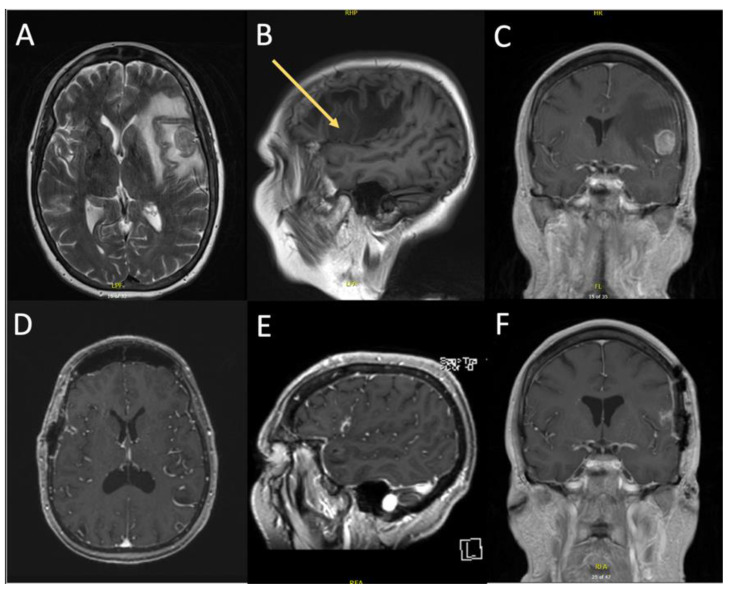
Preoperative MRI (top images) compared with 15-day postoperative MRI (bottom images). Image (A): Axial weighted T2 demonstrating extensive edema associated with lesion seen as a hyperintense signal with midline shift. Image (B): T1 MRI pre-Gd showing a hypointense, rounded, well-defined lesion within pars triangularis of the left inferior frontal gyrus (IFG). Vasogenic edema is visible as a hypointense signal. Image (C): T1-Weighted coronal view with contrast demostrating homogenous enhancement of the lesion and clear visualization of its margins. Ipsilateral collapsed lateral ventricle and midline shift secondary to surrounding hypointense edema is present. Image (D): Weighted T1 post-Gd with complete resection of the lesion. There is a small pneumocranium and a hyperintense signal beneath the craniotomy representing a small subarachnoid hemorrhage. Image (E): T1 weighted MRI showing a small hyperintense signal in the left IFG where the metastasis was previously located. Peritumoral edema is not present compared to before surgery. Image (F): Coronal weighted T1 post-Gd giving another view of the complete resection of the lesion with craniotomy, small hyperintense subarachnoid hemorrhage, and small pneumocranium. The previously collapsed left lateral ventricle is nearly symmetrical due to reduced edema.
